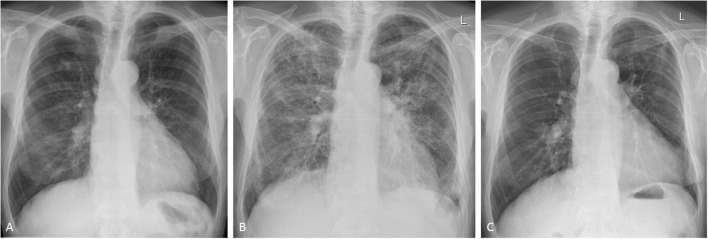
Methotrexate-induced pneumonitis in a 77-year-old man with rheumatoid arthritis. (A) Posterior-anterior chest radiograph immediately before the initiation of treatment. Following 10 days of methotrexate, the patient experienced progressive dyspnea and fever. Follow-up chest radiography showed bilateral heterogeneous opacities in all lung zones. (B) The patient was transferred to the intensive care unit for supportive treatment. High-dose glucocorticoids were administered and gradually withdrawn following clinical and radiological improvement. Initial high-resolution CT scanning showed diffuse infiltrates and bilateral patchy consolidations with only very limited ground-glass opacities (images not shown). (C) Seven months after stopping methotrexate, the changes of pulmonary toxicity had fully resolved.
